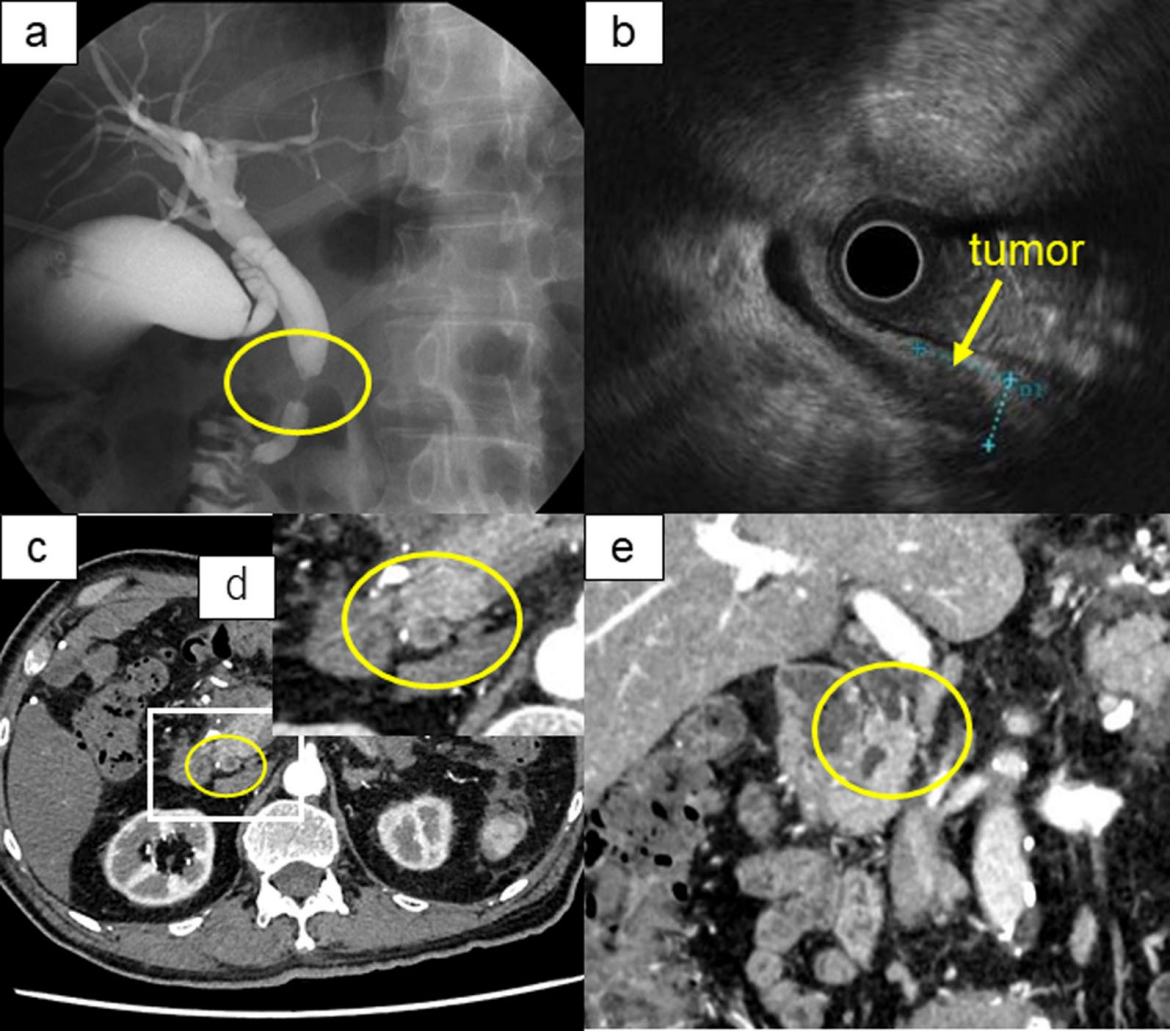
Preoperative examination. a Cholangiography showed severe stenosis of distal bile duct. b EUS showed a hypoechoic mass of 14 × 10 mm in the intrapancreatic bile duct and suggested no invasion of the pancreas and duodenum. c-e Contrast-enhanced CT showed circumferential wall thickening in the intrapancreatic bile duct. *CT* computed tomography; *EUS* endoscopic ultrasound
